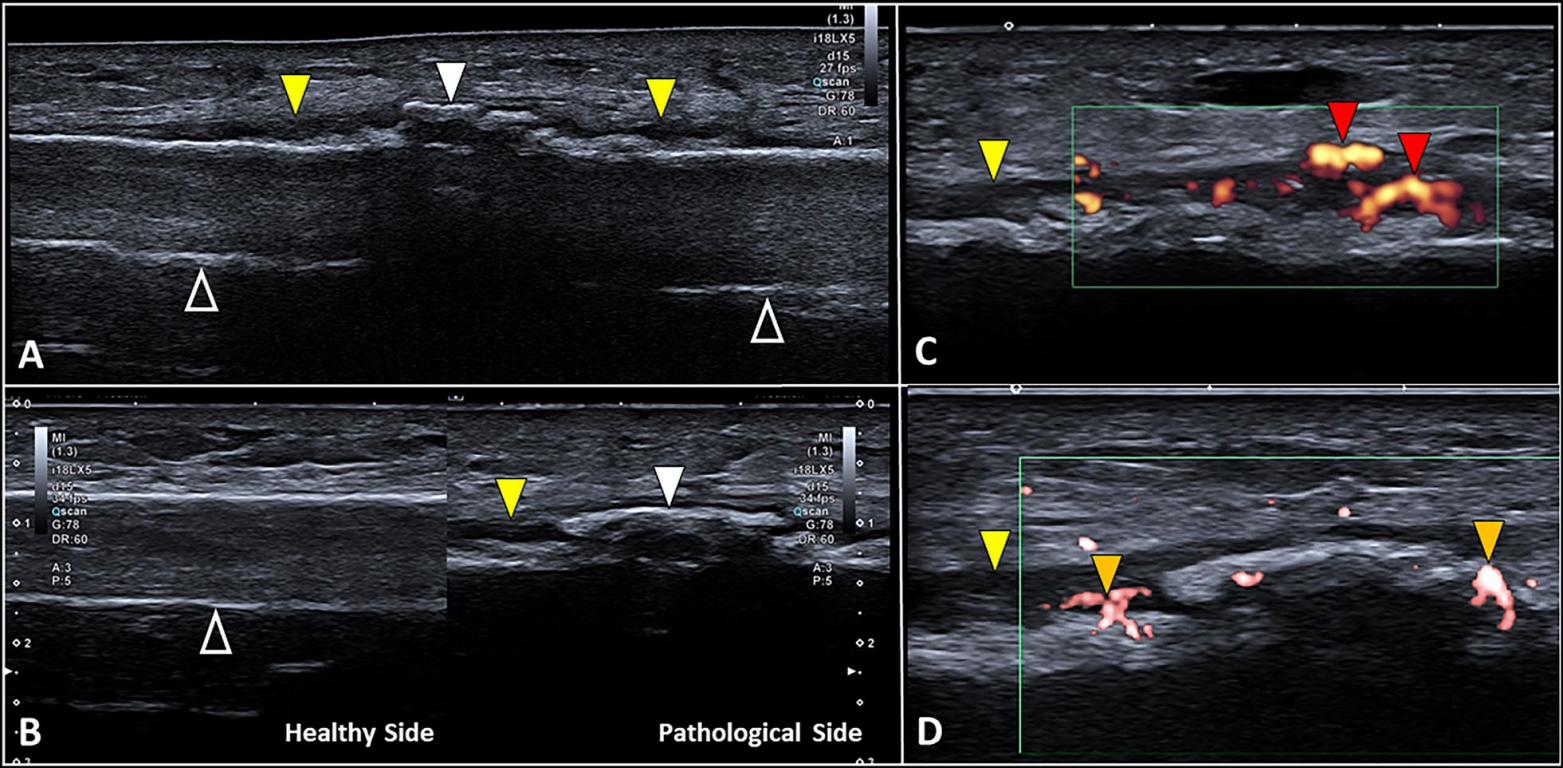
Stress fractures and healing phases. Focal thickening of the periosteum (*yellow arrowhead*), the disappearance of the reverberation artifact (*void arrowheads*) of the cortical bone, and lamellar calcifications (*white arrowhead*) within the periosteum are the most common sonographic findings in the stress fractures (A, B). Using high-sensitive color Doppler to follow up the healing phases of the stress fracture, microvasculature (*red arrowheads*) within the thickened periosteum (*yellow arrowhead*) (C) and penetrating vessels (*orange arrowheads*) crossing through the cortical bone (D) can be observed
